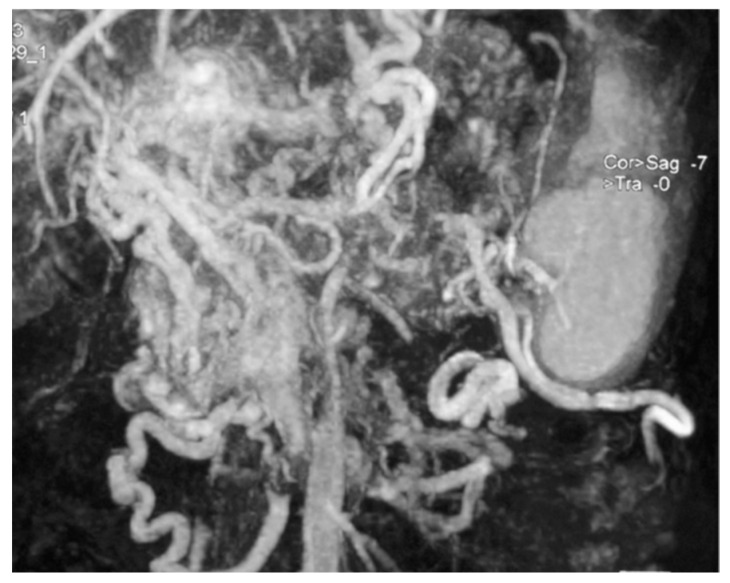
Abdominal magnetic resonance imaging showing several huge mesenteric and left gastric vein compensation collateral circles due to portal vein thrombosis and portal hypertension (case n.6).
